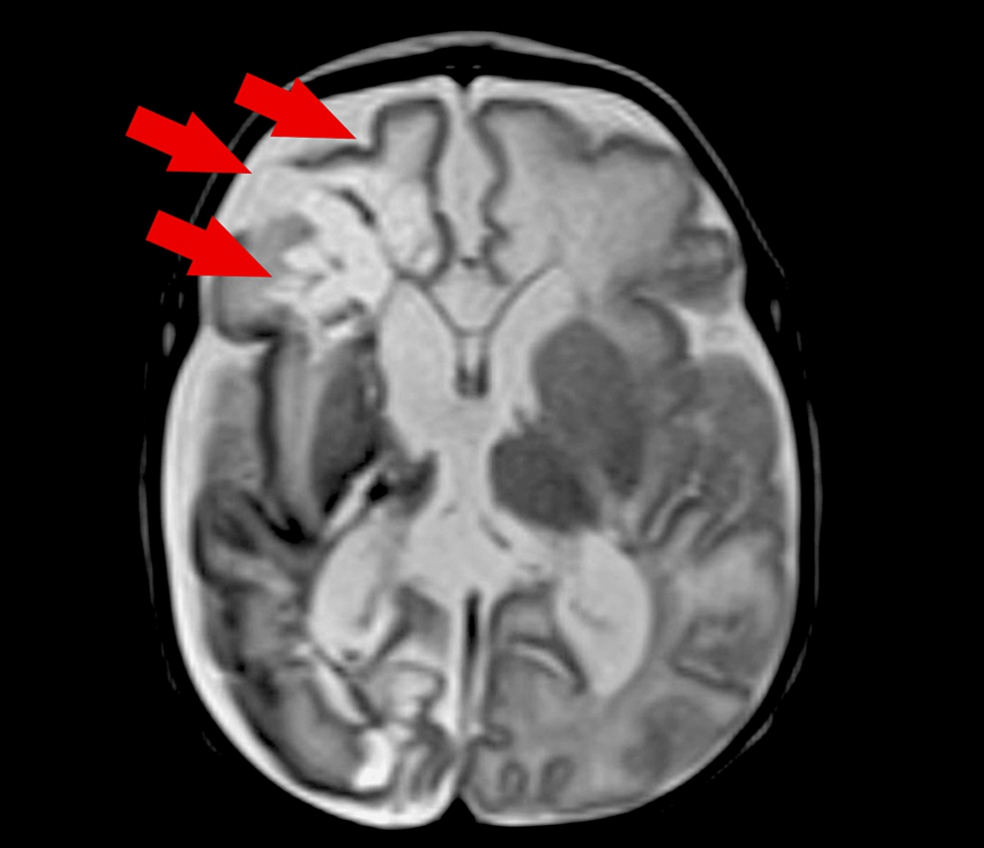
T2-weighted MRI in the transverse plane demonstrating bilateral cystic foci primarily in the distribution of the right MCA on postoperative day 21. MCA: middle cerebral artery
